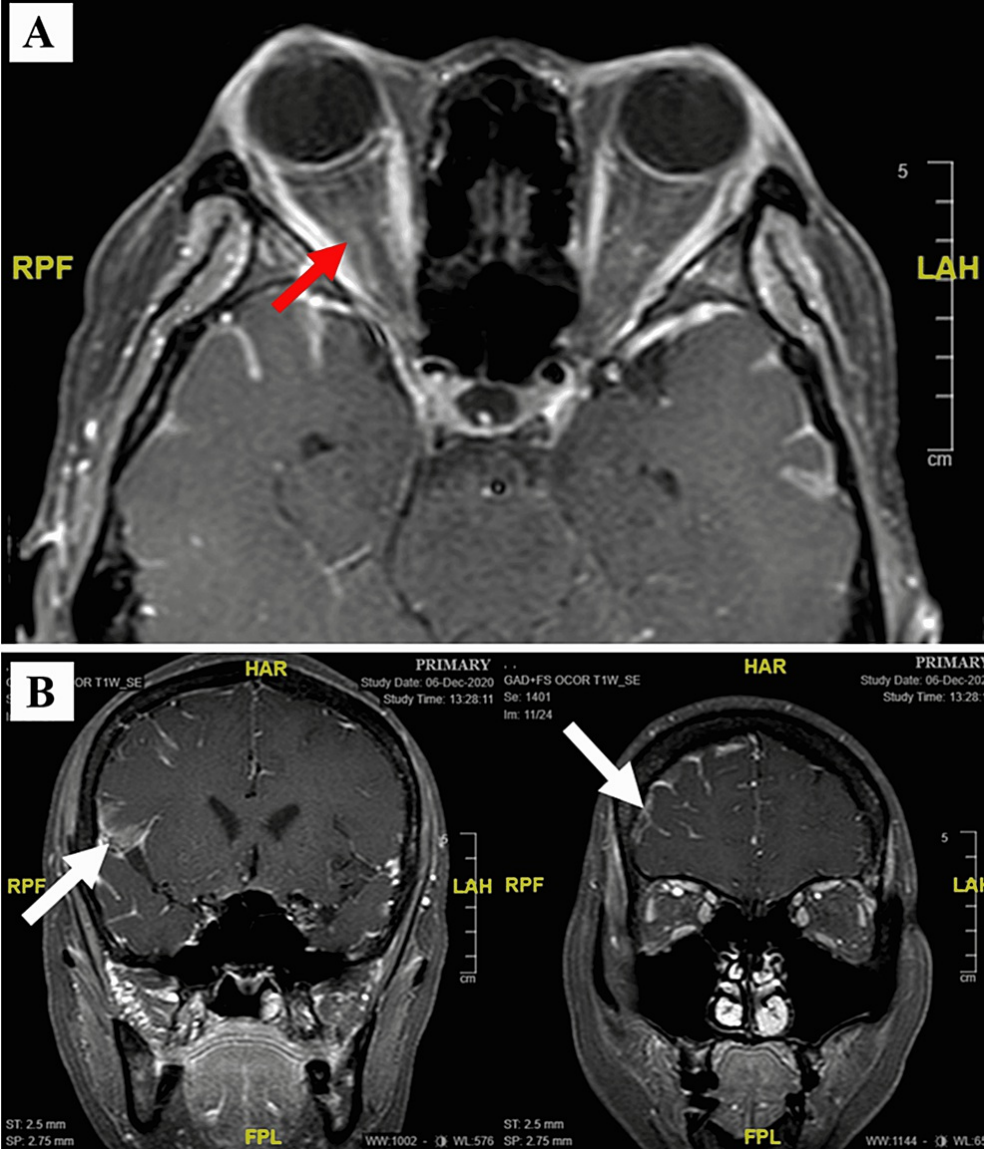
(A) Contrast enhancement of the right optic nerve (red arrow) in gadolinium-enhanced T1-weighted MRI. (B) MRI showing leptomeningeal enhancement (white arrows). MRI: magnetic resonance imaging
